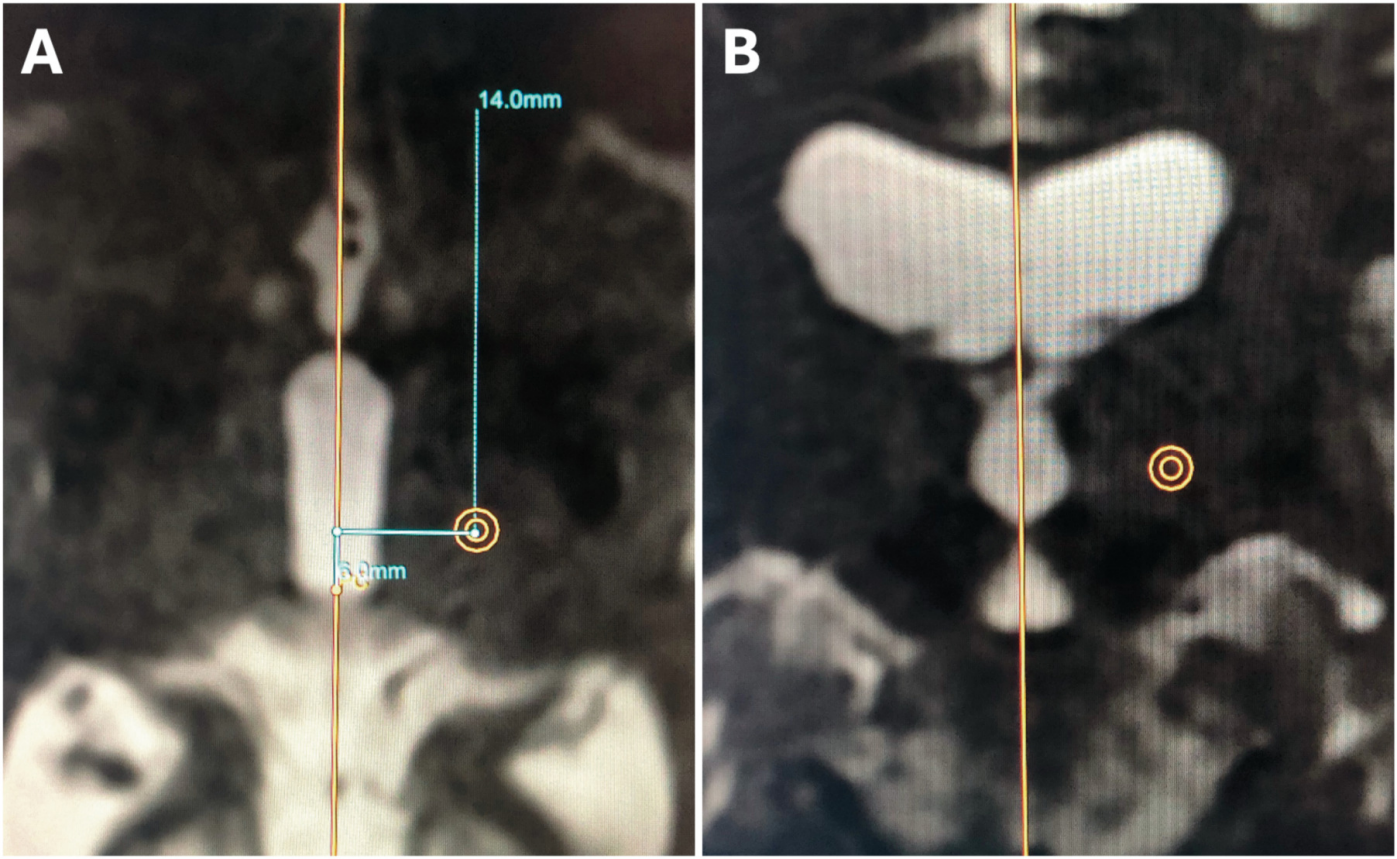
Atlas-based targeting using standard coordinates on a T2w MRI sequence (A: axial viw; B: coronal view). Note the complete lack of anatomical landmarks of any intra-thalamic or extra-thalamic structures.
